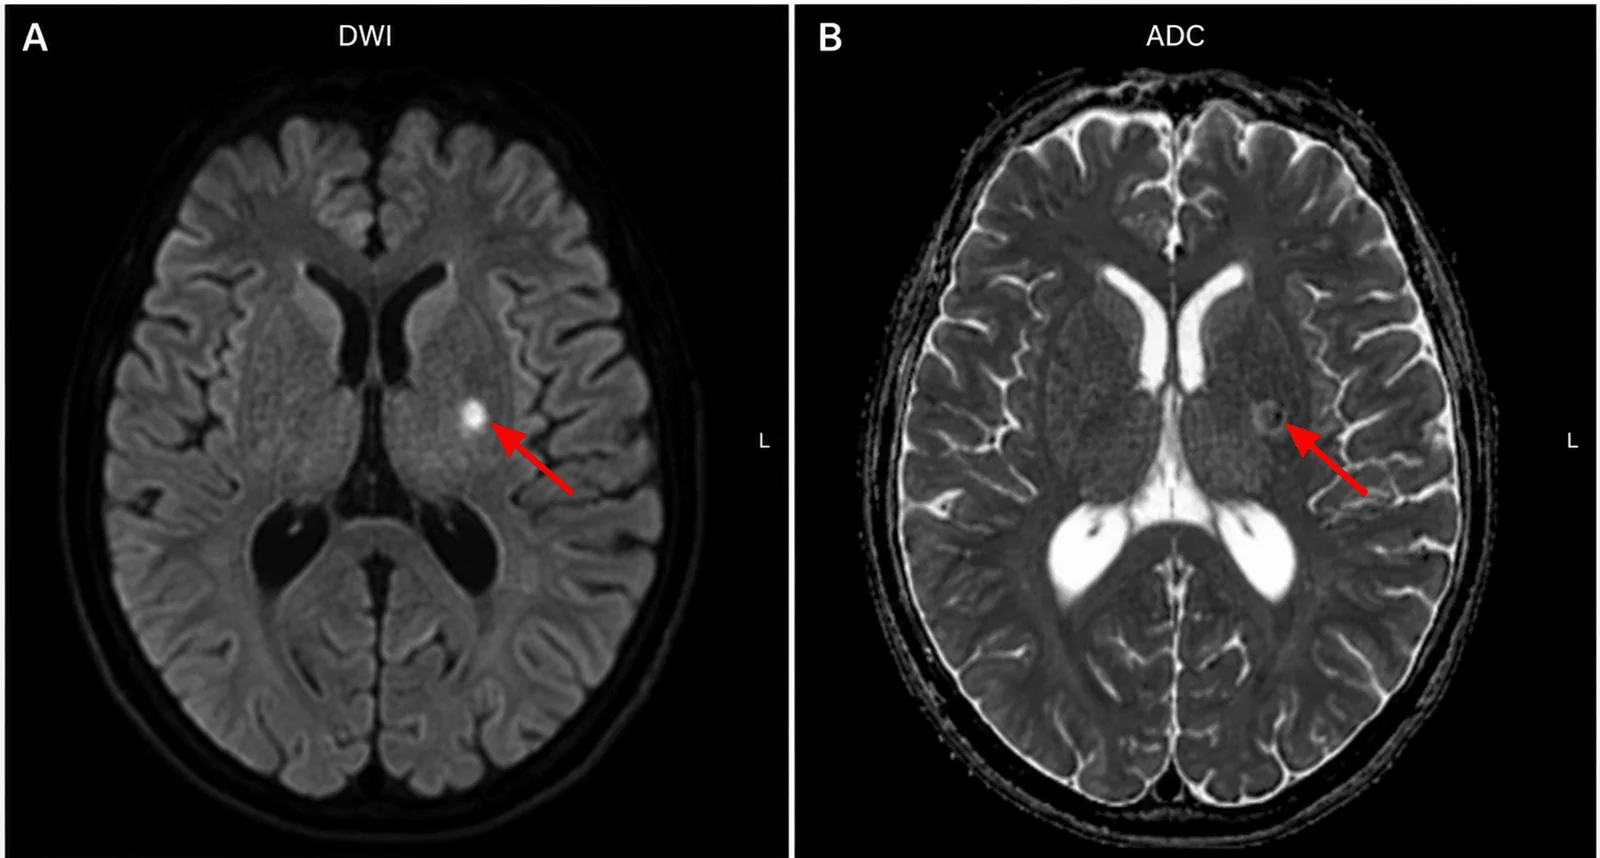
Brain MRI Demonstrating an Acute Left Basal Ganglia Lacunar Infarction Axial brain MRI demonstrating an acute lacunar infarction in the left basal ganglia. (A) Diffusion-weighted imaging (DWI) shows a focal area of restricted diffusion in the left basal ganglia/internal capsule region (red arrow). (B) The corresponding apparent diffusion coefficient (ADC) map demonstrates low signal intensity in the same location (red arrow), confirming true restricted diffusion consistent with an acute lacunar infarction.
